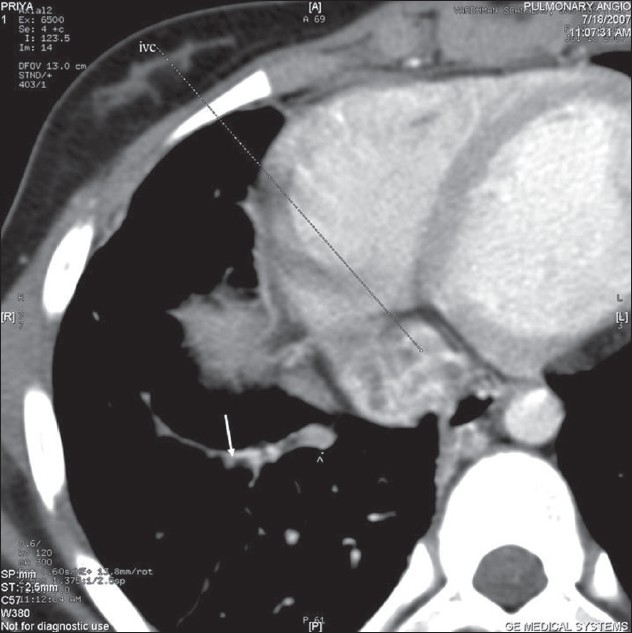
CT thorax showing lower part of the aberrant venous drainage to inferior vena cava (shown as arrow)
